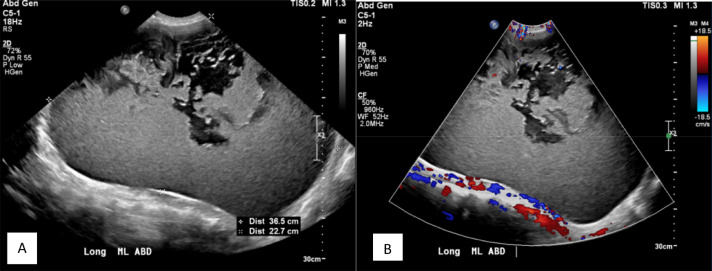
Grey scale (A) and color Doppler (B) longitudinal ultrasound images of the abdomen and pelvis showing a huge mixed echogenicity mass measuring up to 36.5 cm. The mass is predominantly hyperechoic with irregular hypoechoic areas anteriorly and regular outline. Color Doppler image showed no significant flow within the mass, confirming the cystic nature of the mass.
